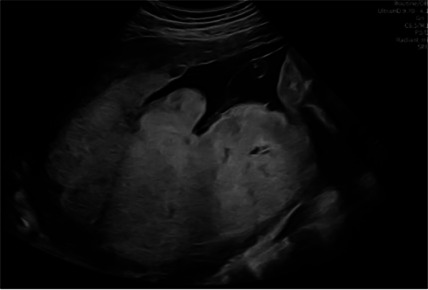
Cardiothoracic ratio (CT ratio)
